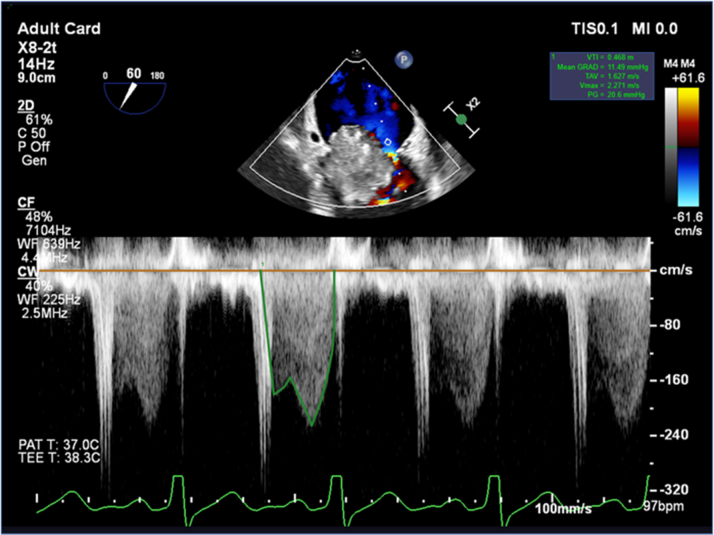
Transesophageal Mean Pressure Gradient of the Mitral Valve
